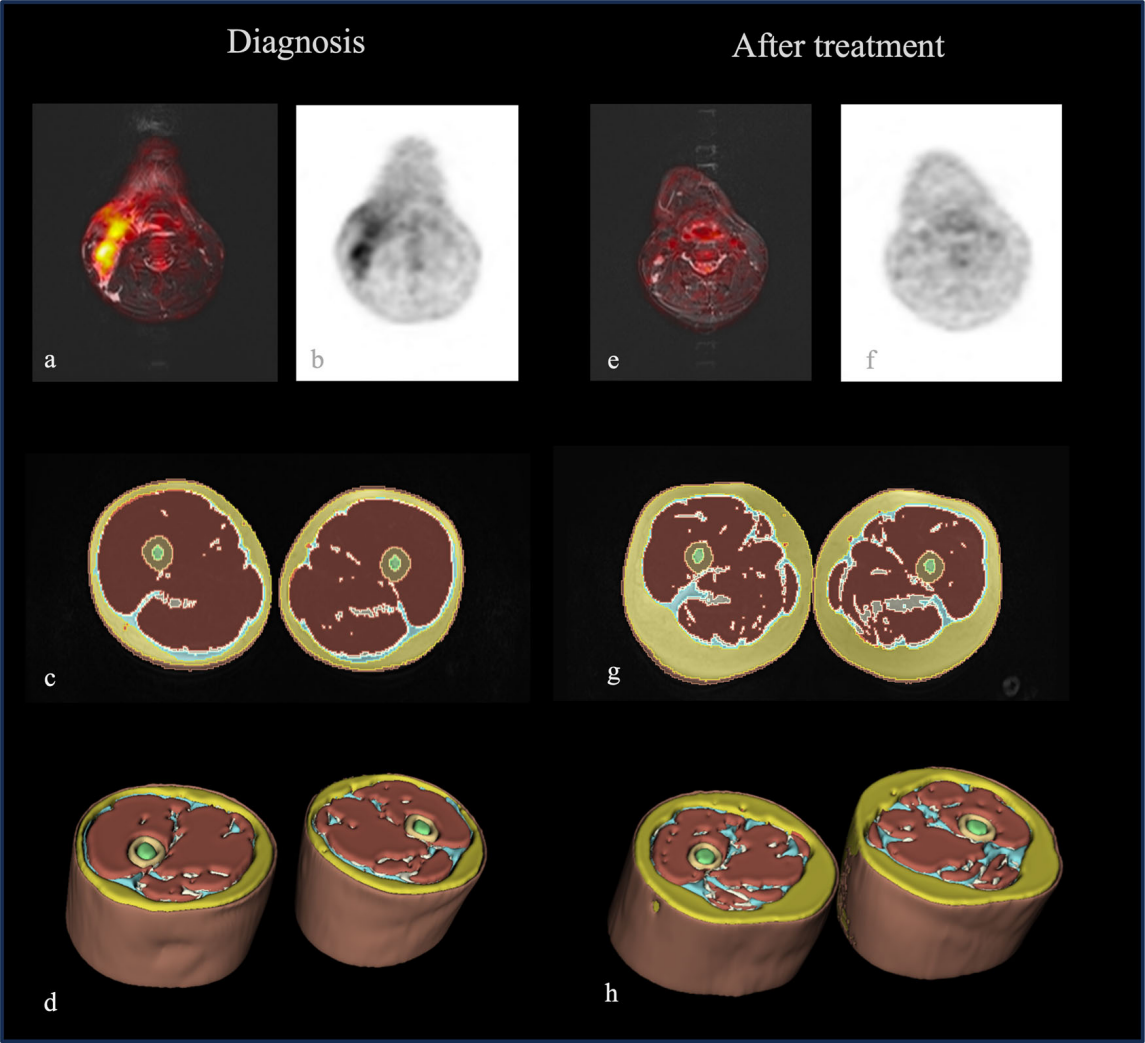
Sixteen-year-old boy with Hodgkin lymphoma examined by [^18^F]FDG-PETMR for staging and restaging. In a and b, the fused axial STIR and the PET map show hypermetabolic cervical lymph nodes. In c and d, the 2D and 3D segmentations of his thighs at staging. After treatment, a full response was achieved as demonstrated by the reduced size and uptake of the cervical lymph nodes (e and f), and in the thighs, a significant increase of the subcutaneous fat, as well as a loss of muscle mass occurred (g and h, respectively)
